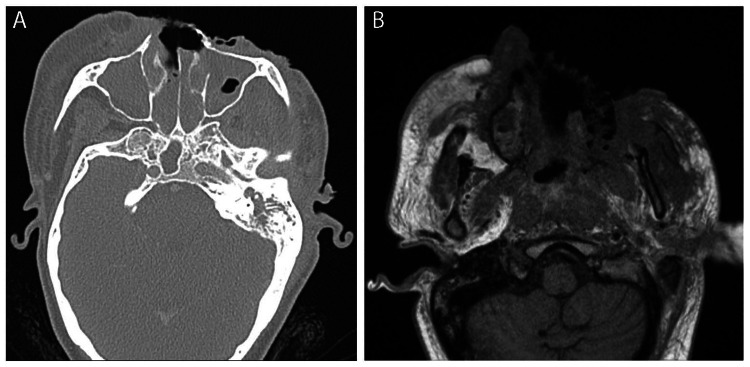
(A) Axial CT at admission showing severe multifocal paranasal sinus opacification with osseous erosions and soft tissue ulceration overlying the anterior and left lateral face with bilateral retroantral, retropharyngeal, and retro-orbital extension. (B) Axial T1-weighted MRI at admission showing extensive soft tissue edema and enhancement with deep overlying ulcerations in the left paramedian face.
